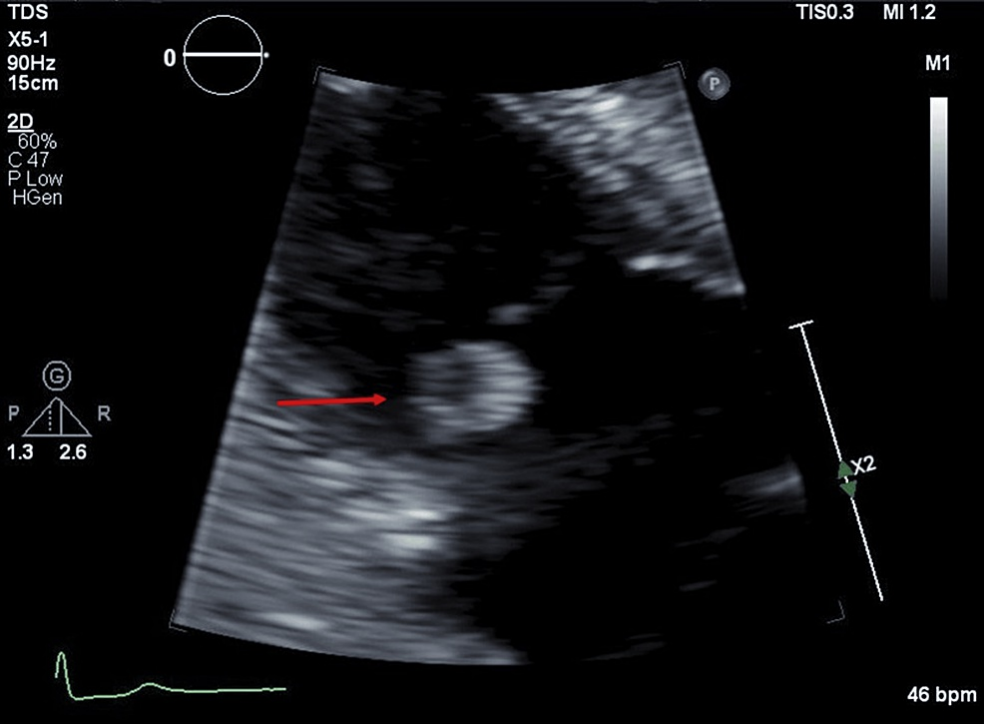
Transthoracic echocardiogram: Right ventricular modified (lateral) apical four-chamber view demonstrates an echogenic mass attached to the anterior tricuspid valve leaflet.
